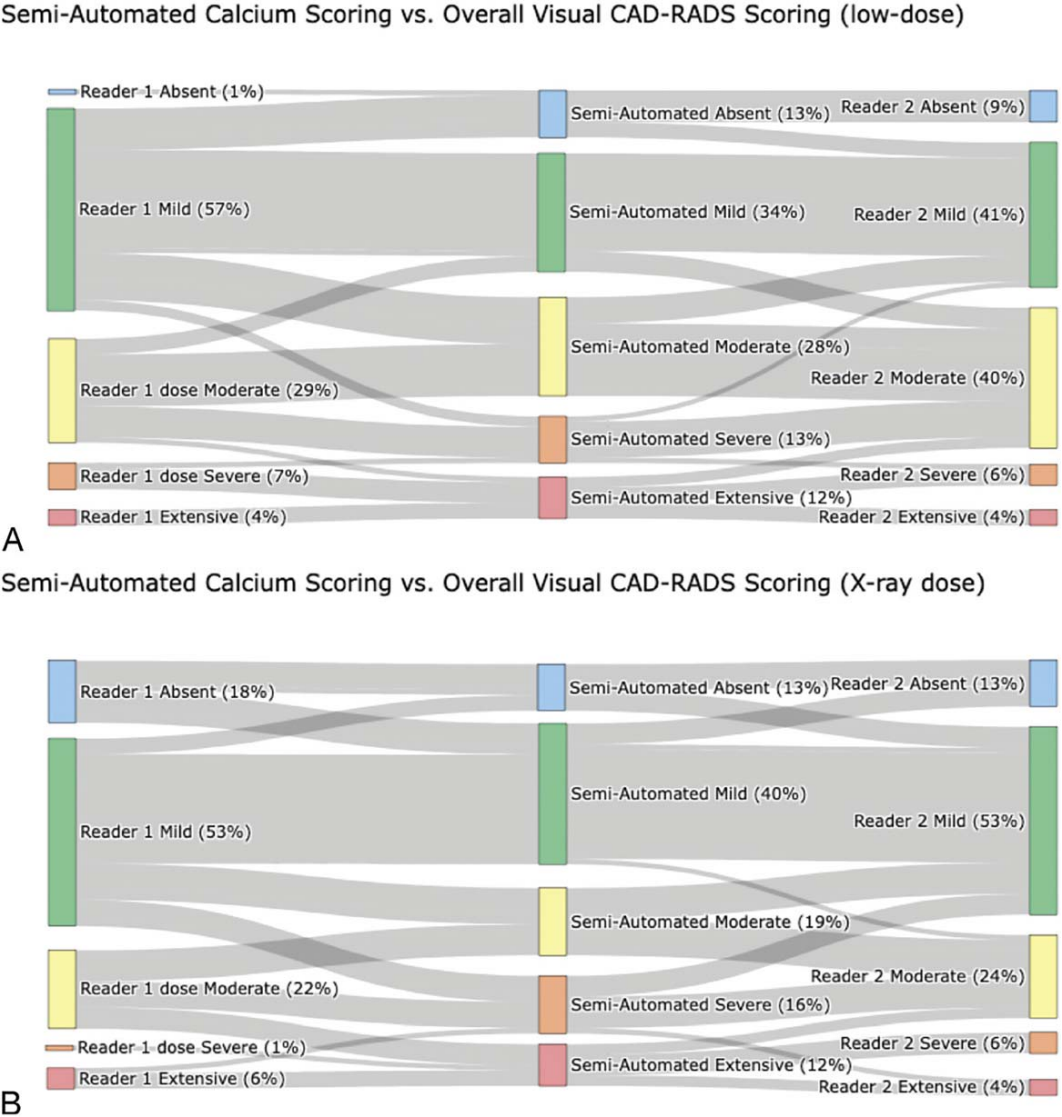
Sankey plots showing the differences in the Coronary Artery Calcification classification between semiautomated scoring and visual scoring at low-dose (A) and radiography-comparable dose (B). Agreement between readers and semiautomated assessment was found to be moderate to substantial for low dose and moderate for radiography dose.
